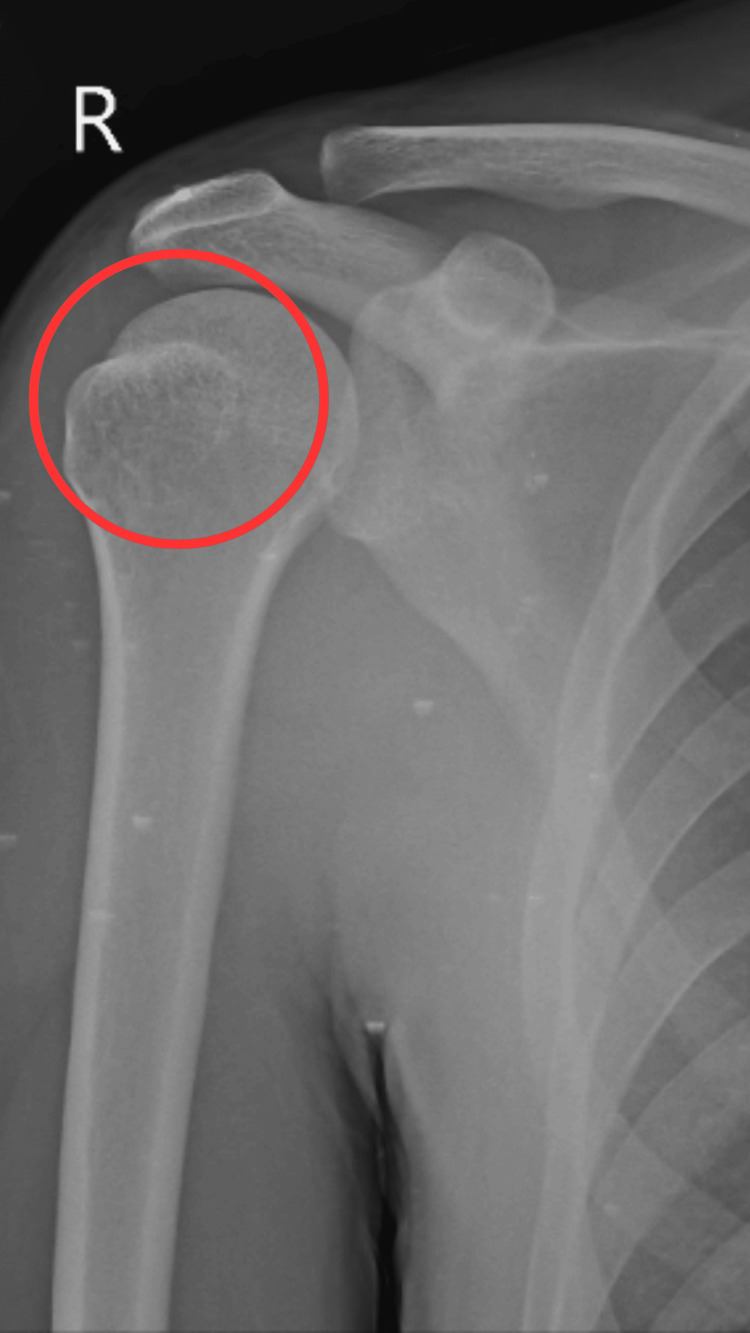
Preoperative X-ray of the right shoulder The red circle indicates a Hill-Sachs lesion in the posterosuperior aspect of the head of the humerus
